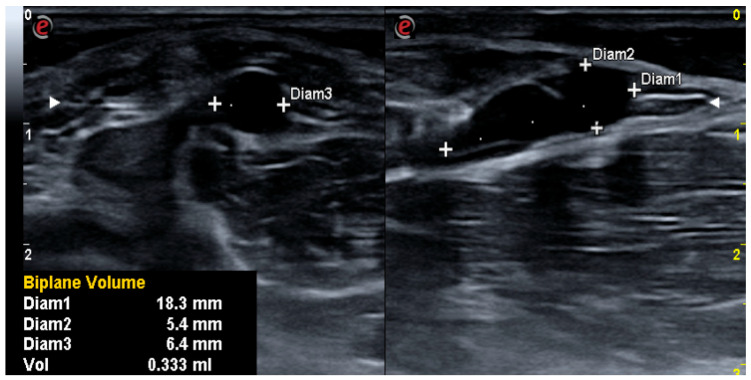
Ultrasonographic images of Baker’s cyst.
